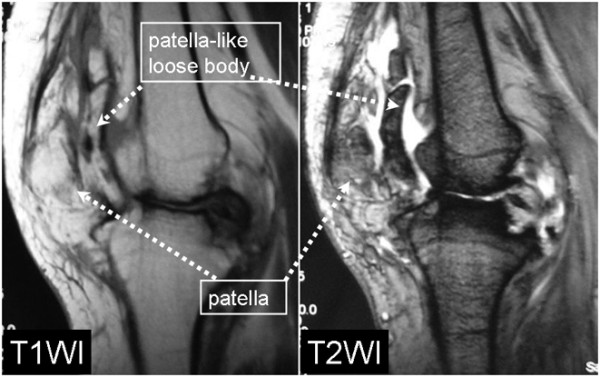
MRI. T2-weighted MRI showed a high-signal-intensity area around the bone fragment, and thus, the fragment was considered to be an intraarticular loose body.
